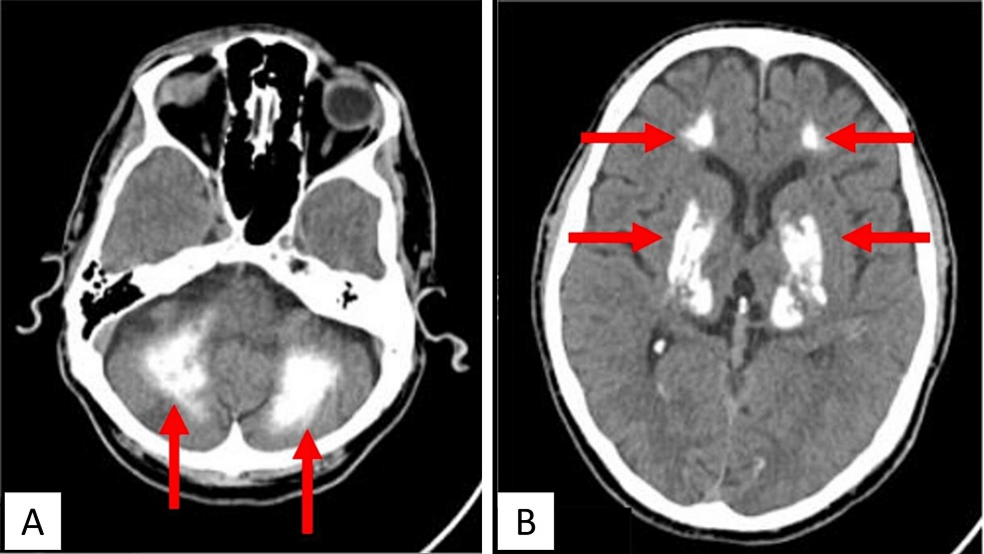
Cranial computed tomography Red arrows show multiple calcifications
